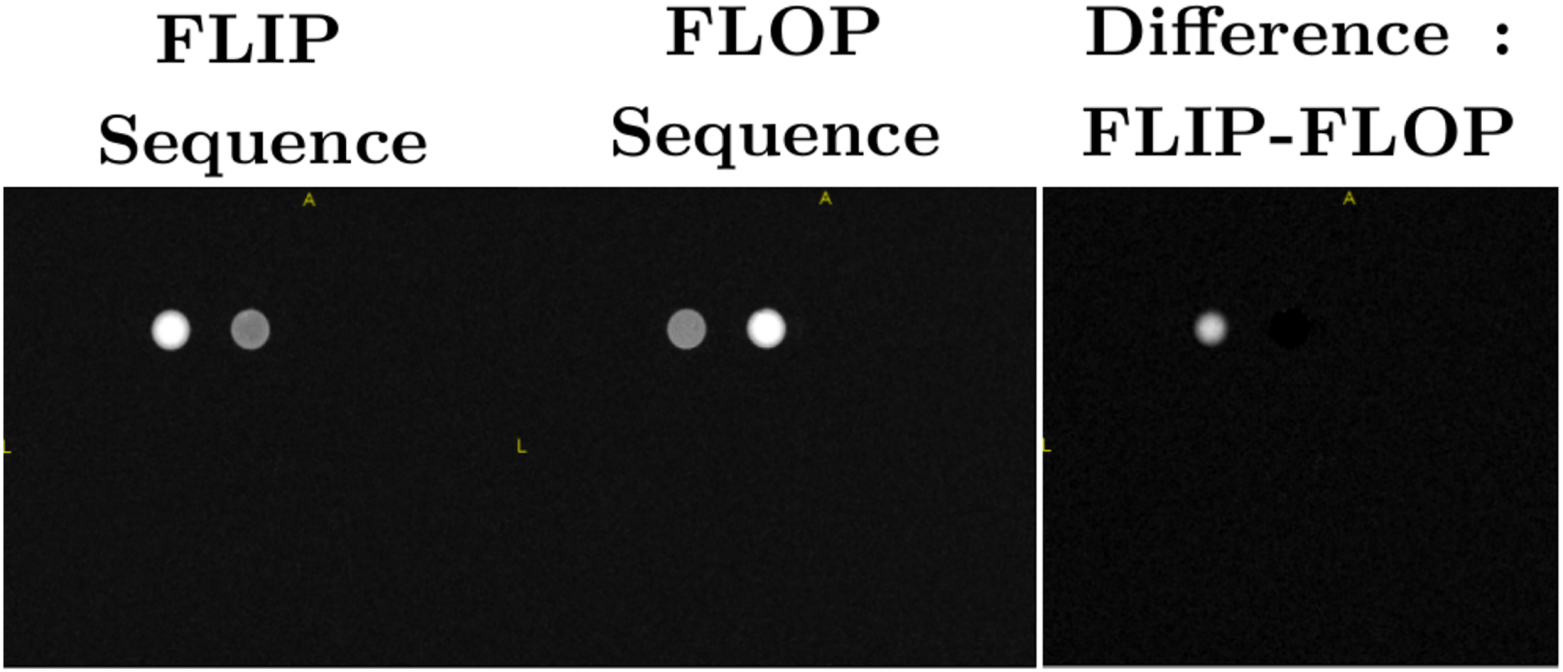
Flip-flop MRI sequence acquisition. From left to right: flip sequence, flop sequence and substracted image (*flip* − *flop*). Experimental conditions: flowing water in a 3mm inner diameter silicone tube, *v*_*mean*_ = 120*μm*/*s*, *T*_*R*_ = *T*_1_/2 = 1.23*s*, *T*_*E*_ = 15*ms*, *FOV*_*READ*_ = 20*mm*, *FOV*_*PHASE*_ = 20*mm*, *N*_*READ*_ = *N*_*PHASE*_ = 128, *T*_*hk*_ = 1*mm*, 4 Slices and *T*_*acq*_ = 2 × 2*min*38.
